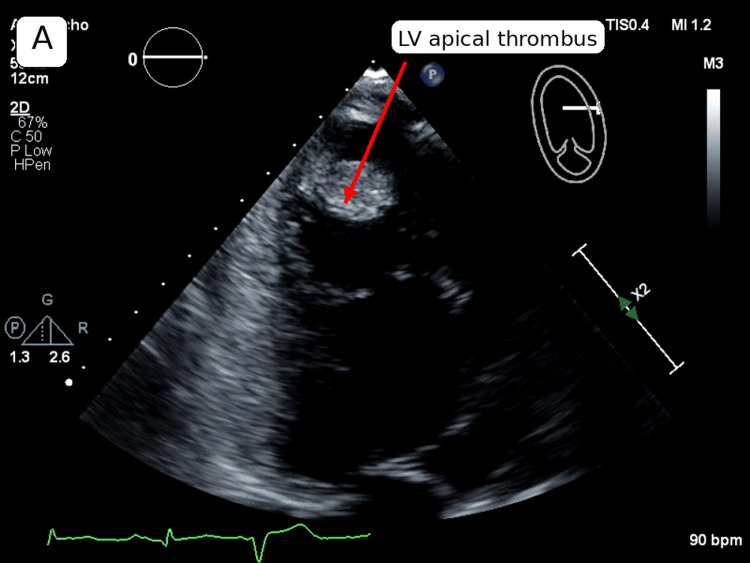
Apical transthoracic echocardiogram demonstrating a mobile left ventricular apical mass (arrow) favored to represent thrombus. In a markedly trabeculated, low-flow apex, adjacent trabeculations and contrast pooling can create a pseudo-mass or pseudo-enhancement appearance that may mimic tumor vascularity on contrast imaging
